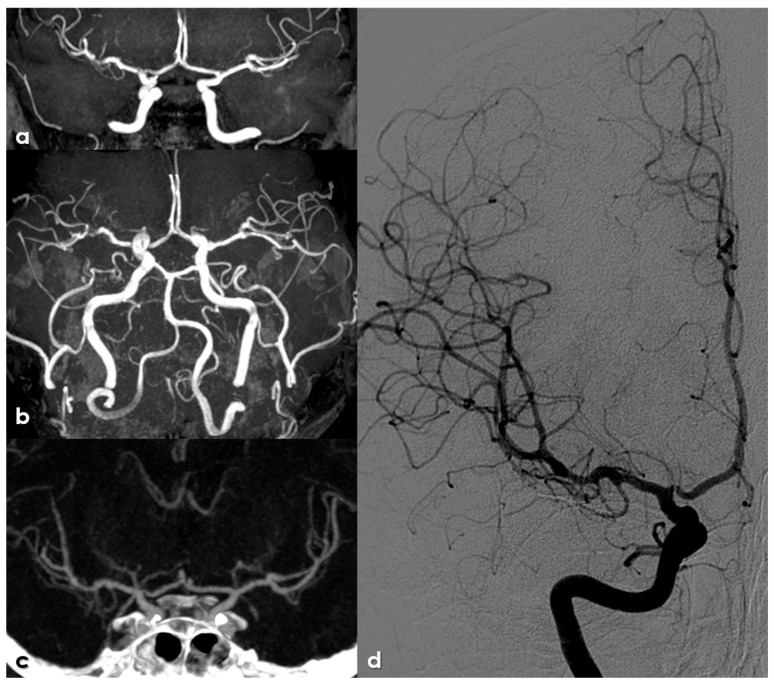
MRA, CTA and DSA of a 58-year-old woman with NF1. Panels (a,b): TOF MRA in coronal view with different obliquations, showing a flow gap in the right distal M1 MCA and in the left posterior M2 MCA branch, suggestive of stenosis. Panel (c): CTA (MIP/MPR protocol) in coronal view, showing very mild arterial stenosis in the same sites. Panel (d): DSA (posterior–anterior view from right ICA injection, showing a sequential mild stenosis in the right distal M1 MCA and a focal stenosis in the right A1 ACA.
